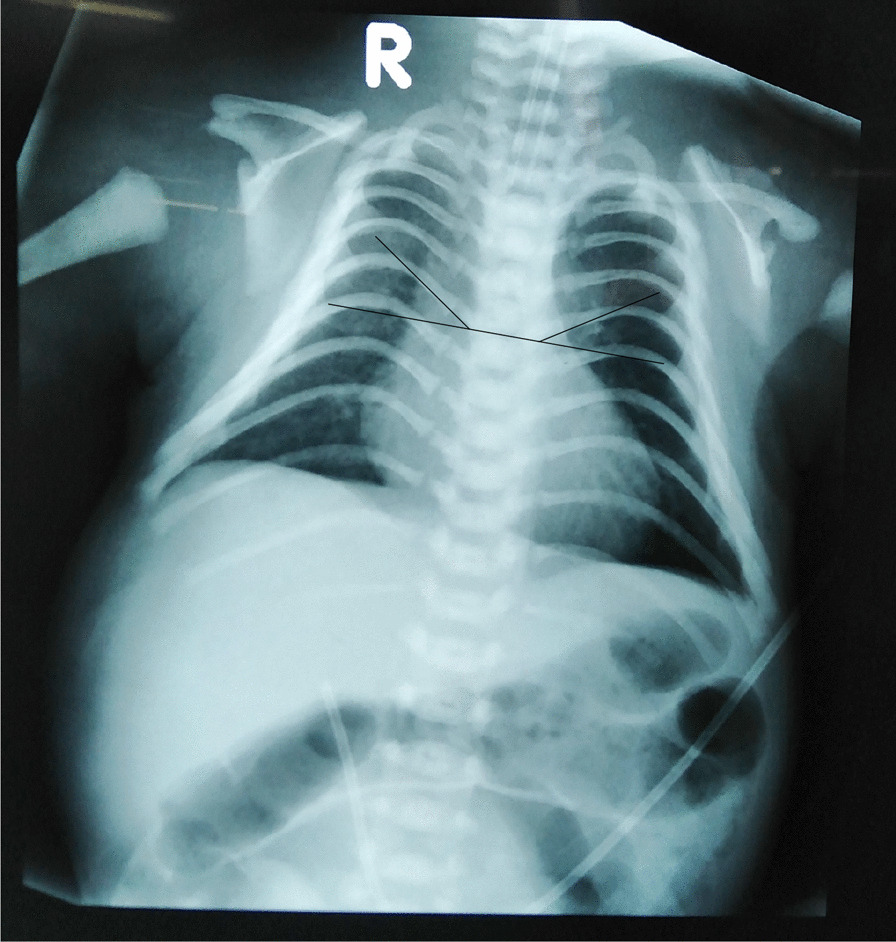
Chest x-ray showing coat-hanger appearance of the rib cage with increased coat-hanger angle (33° on the right side and 31° on the left side). In addition, the ratio between mid-to-widest thoracic diameter is significantly decreased to 68%
